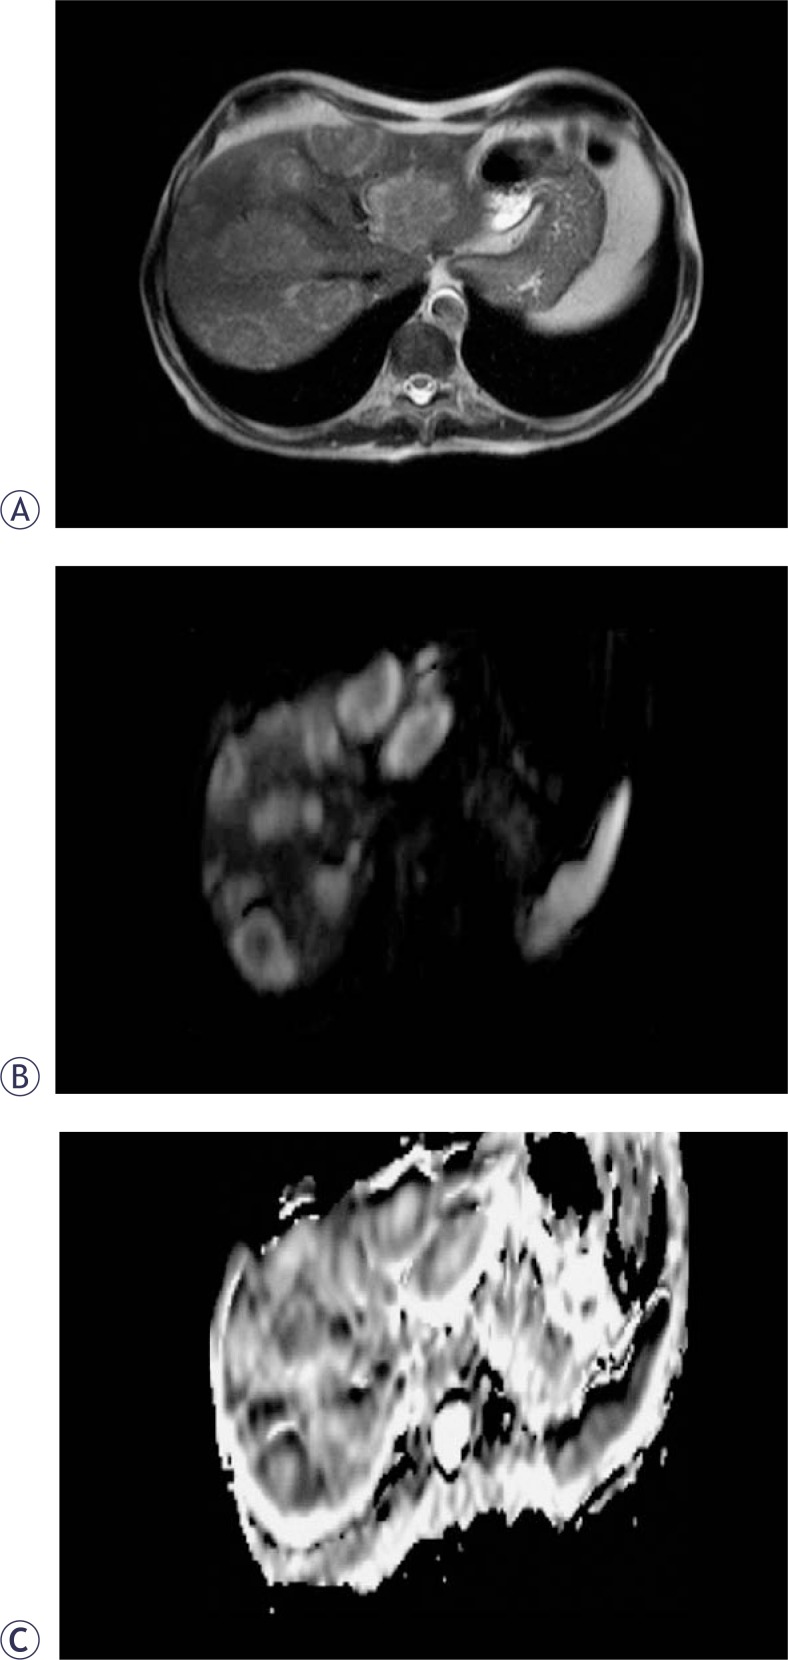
69-year-old woman with multiple metastases of the liver. Axial T2-weighted TSE (A) MR images show multiple metastases in the right and left lobe of the liver. These metastases appear hyperintense compared to the liver on the diffusion-weighted image with b factor 1000 sec/mm^2^ (B). ADC map. Lesion-to-liver ADC ratio=1.5 (C).
